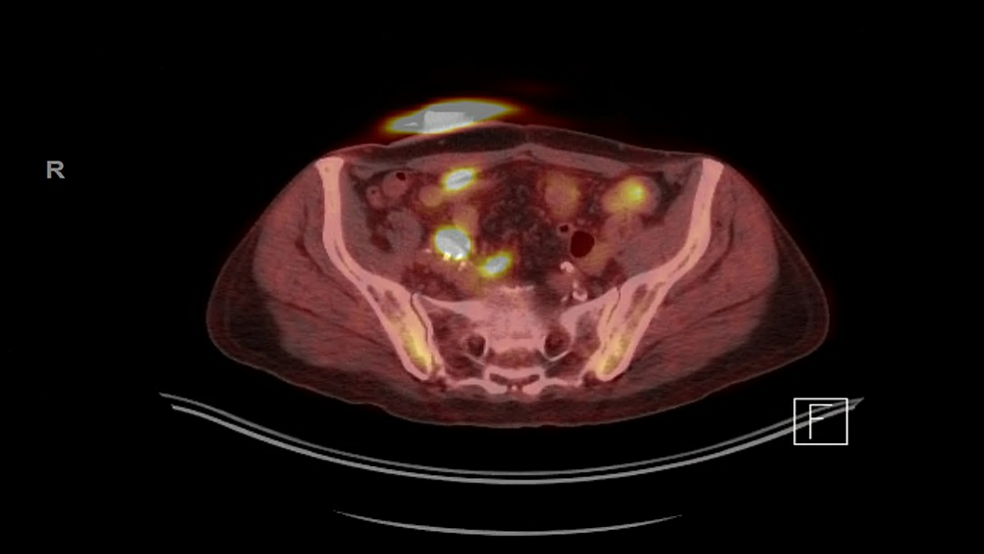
PET/CT image showing multifocal FDG-avid lesions. PET/CT: positron emission tomography/computed tomography; FDG: fluorodeoxyglucose
